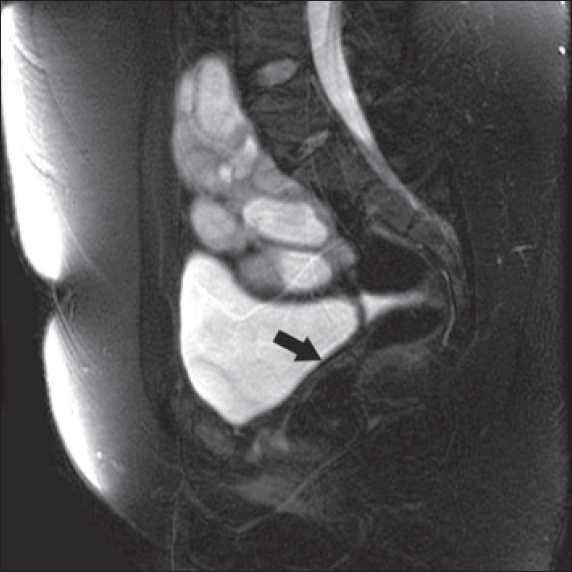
MRI showing pelvic kidney and absent uterus (Arrow)
